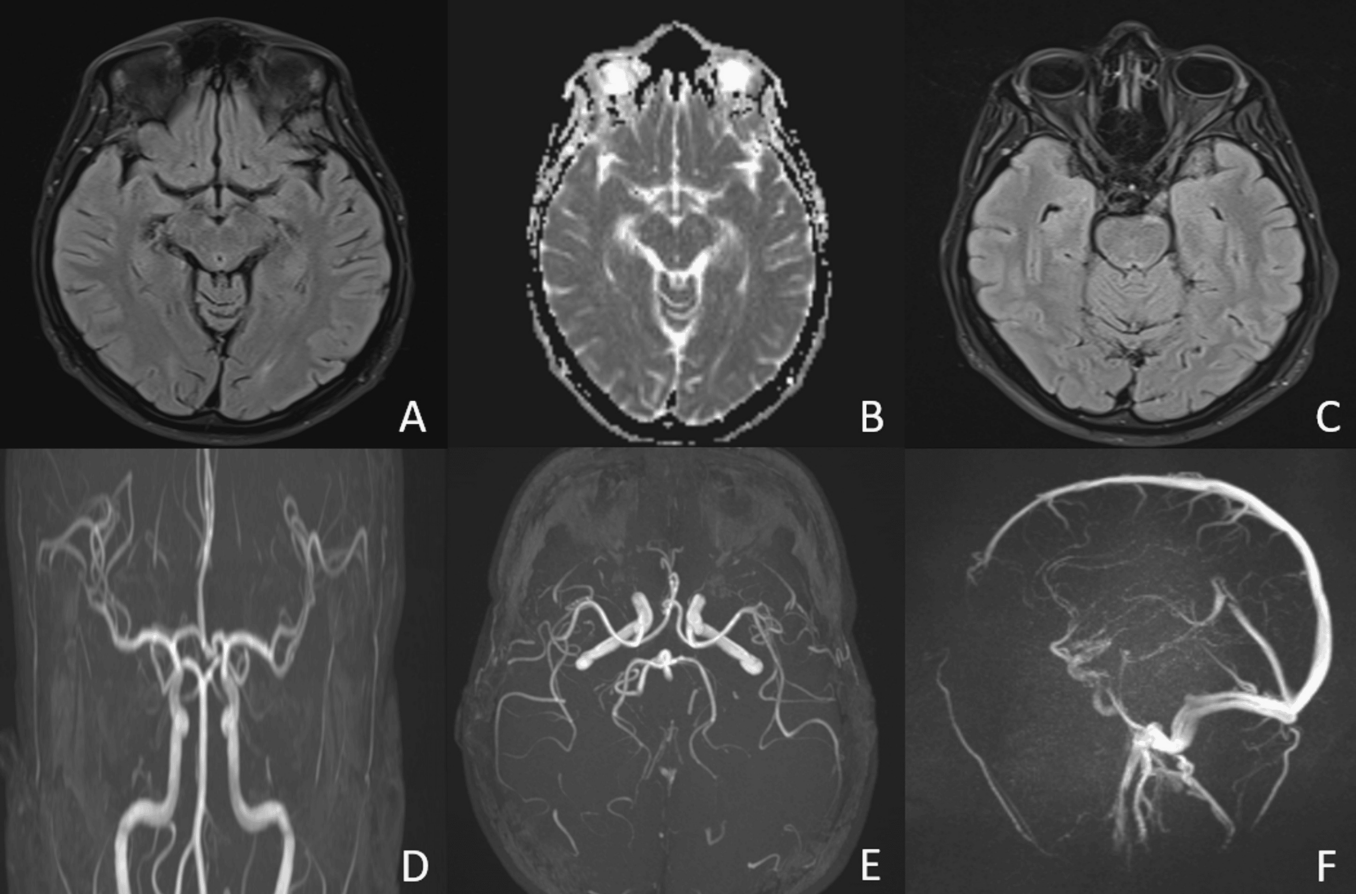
Cerebral MRI with arterial and venous angiographic sequences (A) Axial FLAIR image showing no parenchymal abnormalities; (B) Diffusion-weighted imaging showing no restricted diffusion; (C) Axial high-resolution T2-weighted image focused on the oculomotor nerve, showing no abnormality of its intracanalicular segment; (D) Time-of-flight MR angiography demonstrating normal intracranial arterial anatomy; (E) Maximum intensity projection of TOF MRA showing no aneurysm of the posterior communicating arteries; (F) Sagittal angiographic reconstruction showing no vascular abnormality FLAIR: fluid-attenuated inversion recovery; TOF: time of flight; MRA: magnetic resonance angiography
